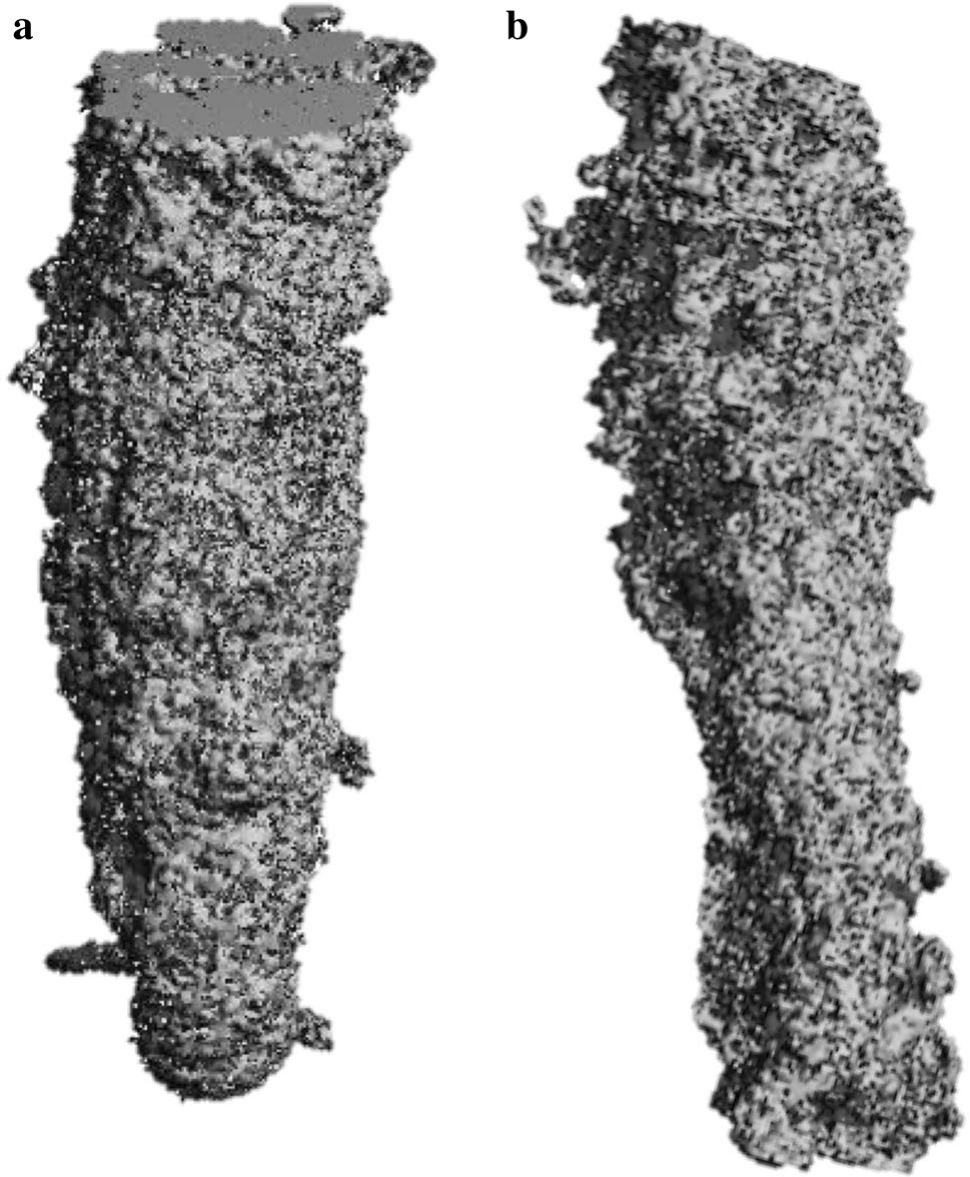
3D reconstruction through micro CT imaging of the bone cavity after a conventional anchor and b all-suture anchor pullout
